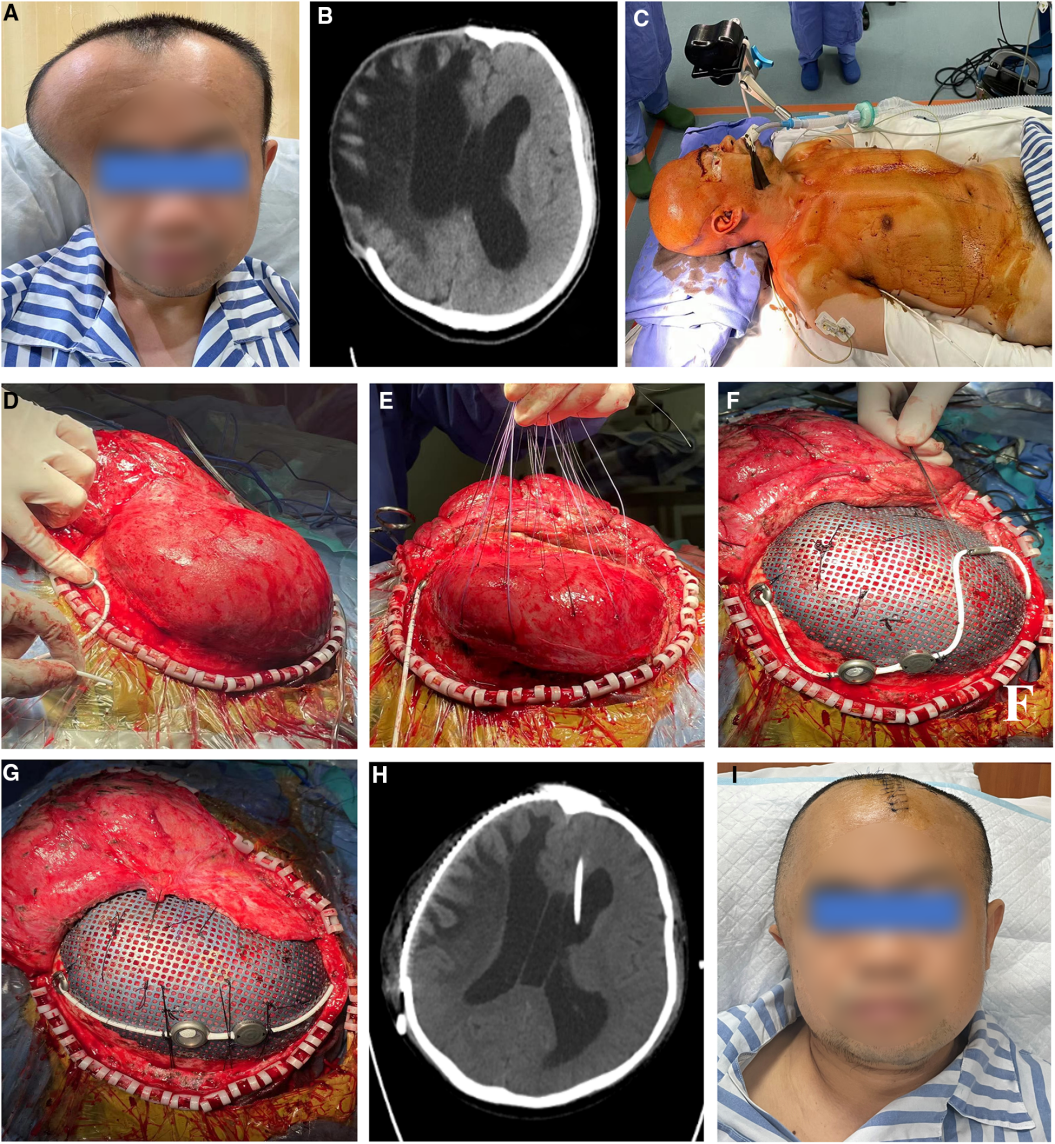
(A) severe encephalocele of the right frontotemporal region was observed. (B) Head CT scan revealed enlargement of the ventricular system. (C–G) Because of severe brain bulging, VP shunt was first implanted to release the CSF. The frontal ventricular puncture at the frontal bone was guided by the neuronavigation system. The parts of shunting devices were connected to the titanium mesh by an improved method. (H) Postoperative head CT showed no subdural/epidural fluid collection or subdural/epidural/cerebral hematoma. (I) The patient recovered rapidly and was discharged a week later. VP, ventriculoperitoneal; CSF, cerebrospinal fluid.
